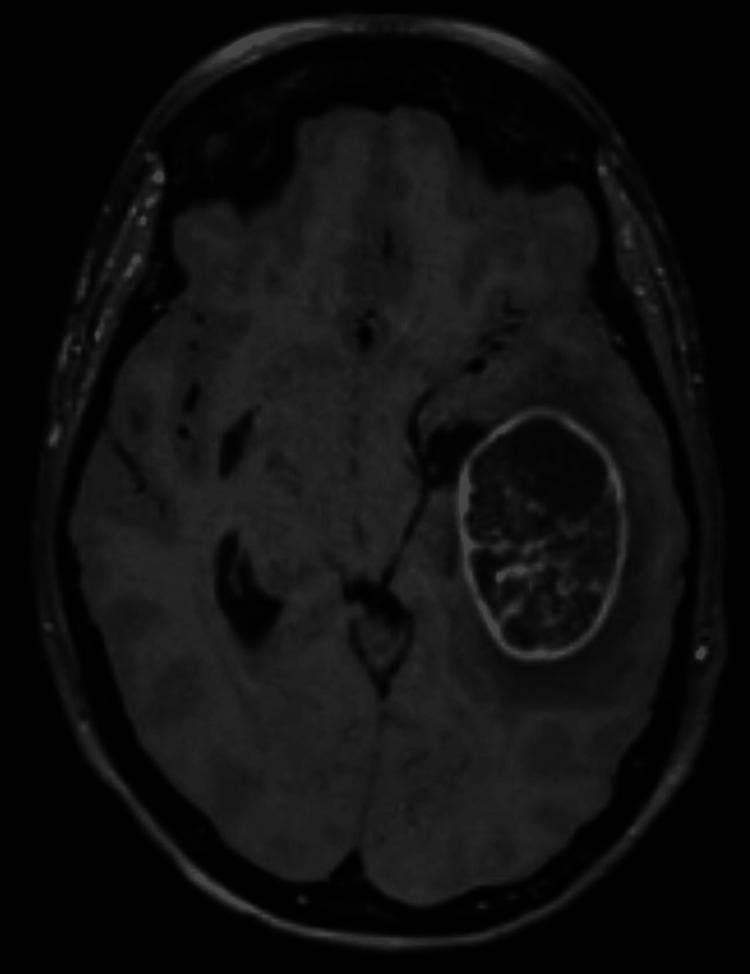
MRI cerebrum T1 sequence with contrast six days after starting chemoimmunotherapy showing left temporal lobe brain metastasis with midline shift and uncal herniation. The hypodensity on the ventral part of the lesion is suggestive for central necrosis since it does not enhance. This is suggestive for tumor response.
